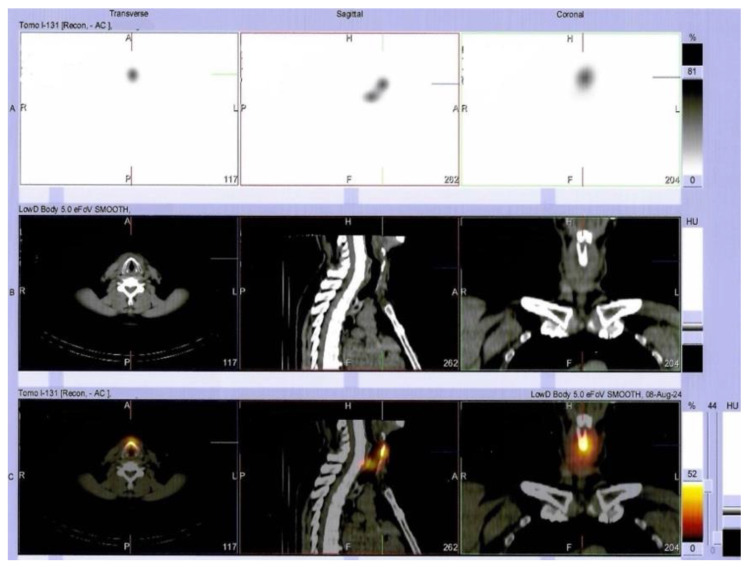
Additionally, single-photon emission-computed tomography combined with CT (SPECT/CT) was performed (immediately after radioiodine administration) and confirmed areas of intense uptake at the level of both the thyroid lobes and pre-tracheal, corresponding to iodine fixating thyroid remnants that during scintigraphy (upper row (A): SPECT image; middle row (B): CT image, lower row (C): SPECT/CT fusion image).
